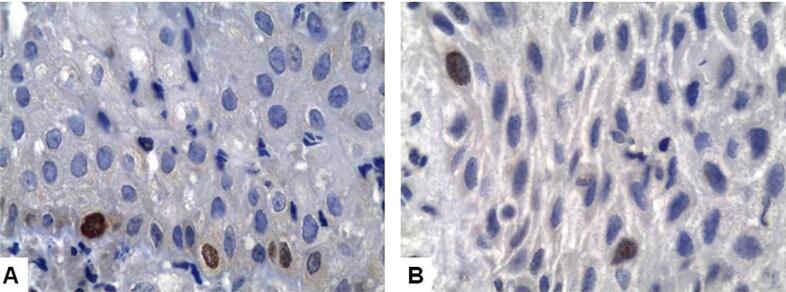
A and B - Nuclear Ki-67 expression in the epithelial lining of two periapical cysts (Immunoperoxidase, 1000x)
